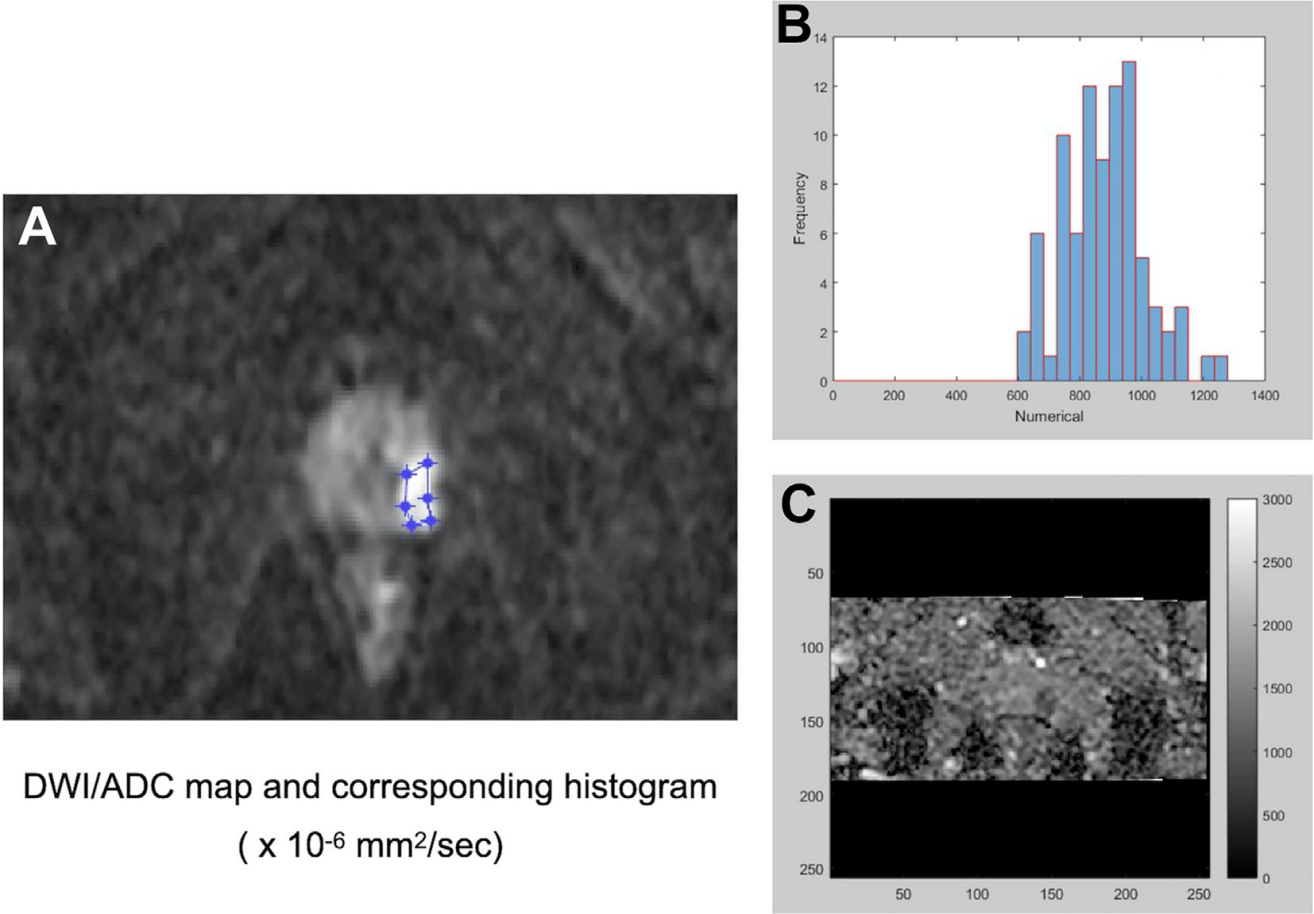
A representative PCa with a preoperative Gleason score of 3 + 3 for the histogram analysis of DW imaging measures. After identifying the dominant tumor nodule in the prostate gland, a region of interest (ROI) was delineated manually on a conventional DWI image (b = 1500 s/mm^2^) (A) to obtain an ADC map and the corresponding histogram (B) of the ADC map (C).
